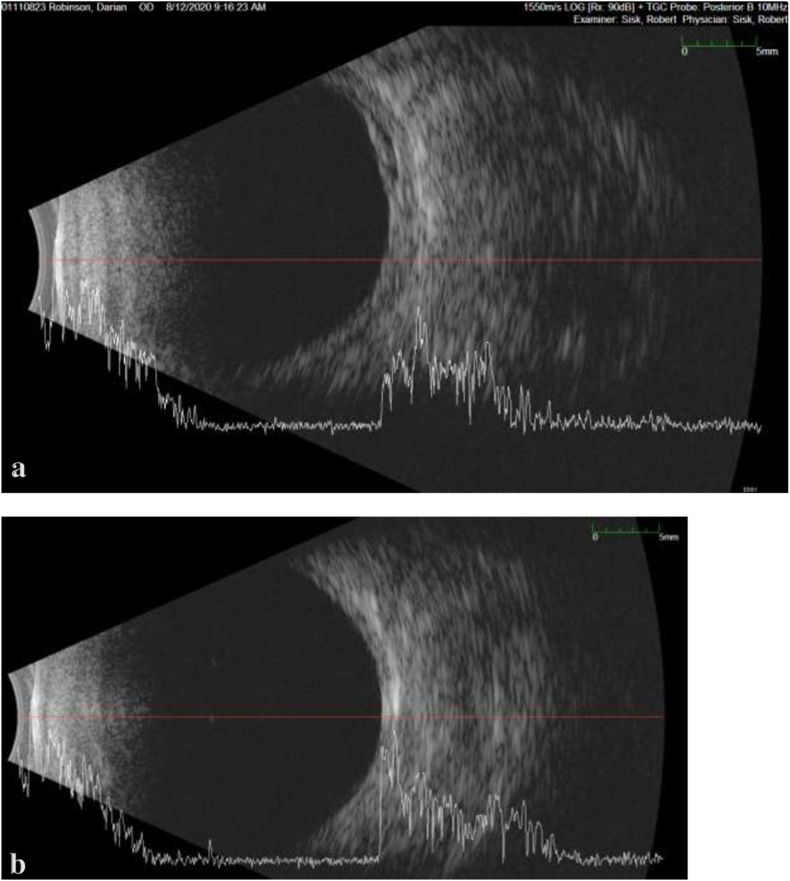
B scan ultrasonography. a) fluid in the suprachoroidal space of the right eye indicating a choroidal detachment, choroidal thickness measured 2.3 mm. b) unremarkable left eye with choroidal thickness of 1.04 mm.
